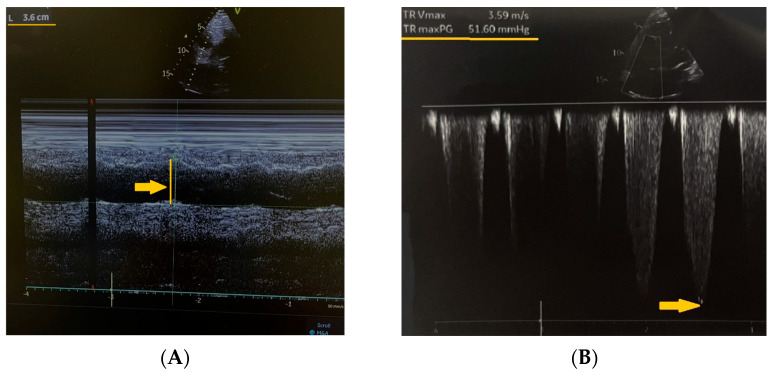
(A,B) Echocardiography revealing a dilated right ventricle 36 mm in the parasternal long-axis view and a maximal tricuspid valve gradient of 51.6 mmHg measured by continuous wave Doppler (yellow arrows).
